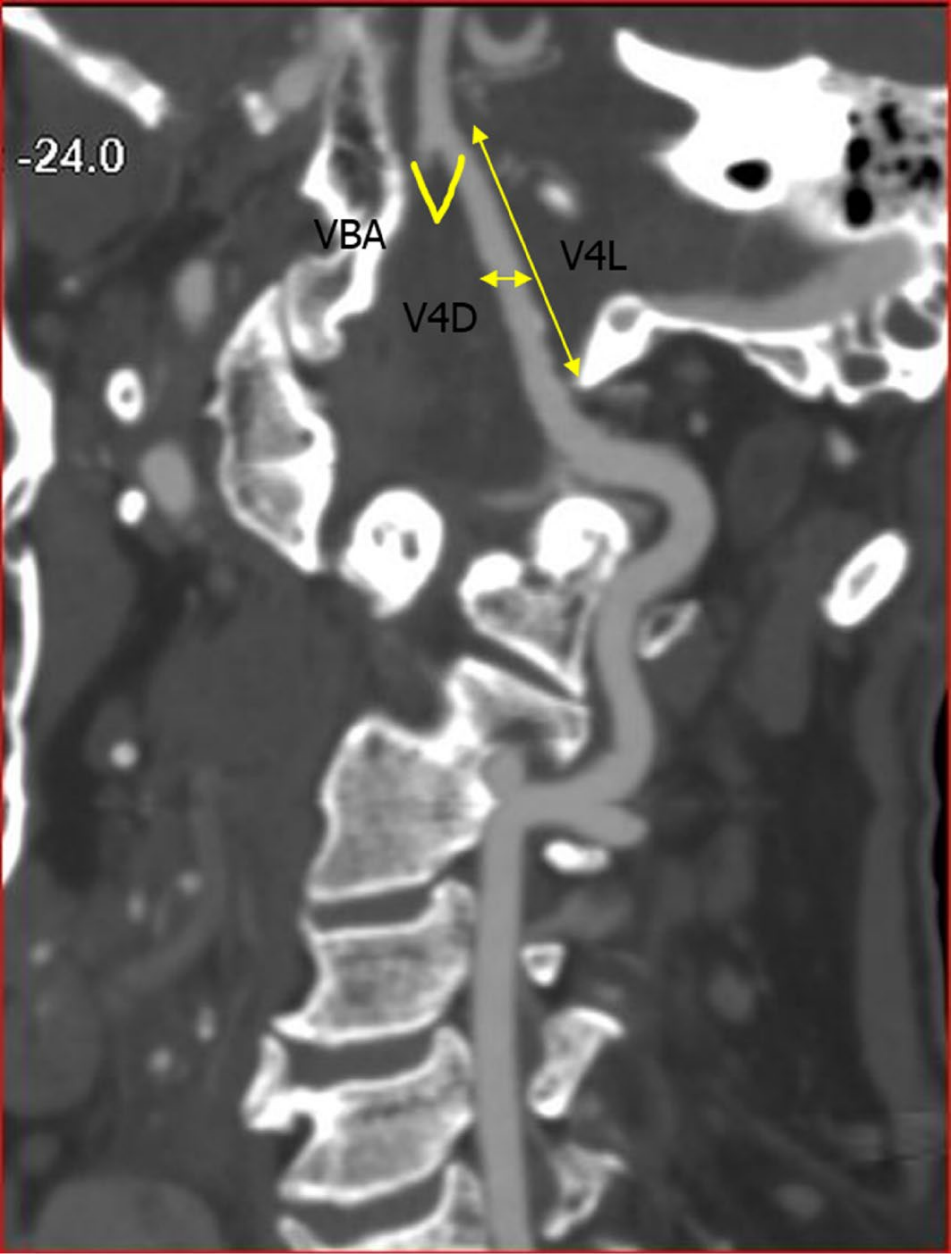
Oblique view of CTA image, showing the V3 and V4 segments of the VA. V4L length of the V4 segment; V4D Diameter of the V4 segment; VBA the angle at the vertebrobasilar junction.
